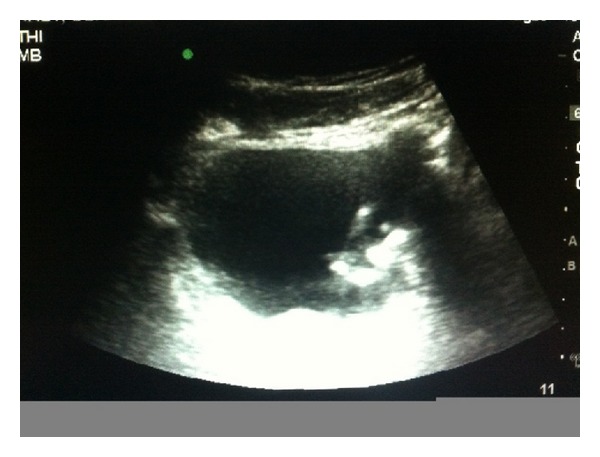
Bedside ultrasound demonstrating hyperechoic object within the bladder.
